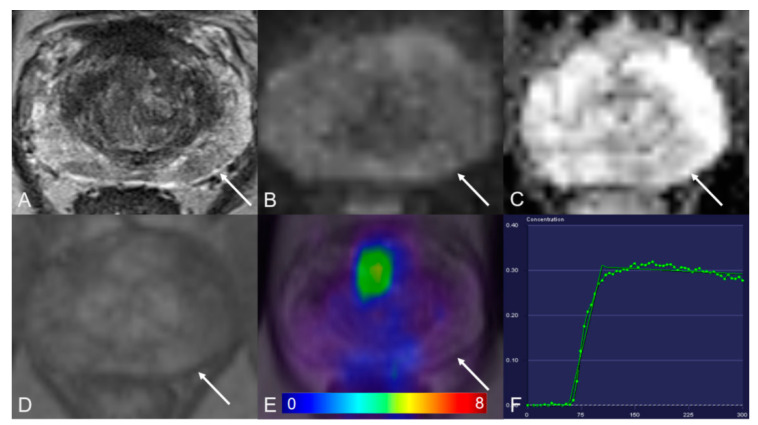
Peripheral zone with a PI-RADS 3 lesion. (A) Axial T2WI shows hypointense. (B) DWI (b = 1000 s/mm^2^) shows a mild hyperintense signal. (C) ADC map image presents a moderate hypointense signal below the background. (D) Early dynamic contrast-enhanced image presents no early enhancement within the lesion. (E) [^68^Ga]Ga-PSMA-11 PET/MRI fusion image shows no positive [^68^Ga]Ga-PSMA-11 uptake. (F) DCE-MRI time-intensity curve demonstrates plateau enhancement. AT: 0.98 min; TTP: 0.67 min; W-in: 0.26; W-out: 0.003; PEI: 0.20; iAUC: 0.13.
